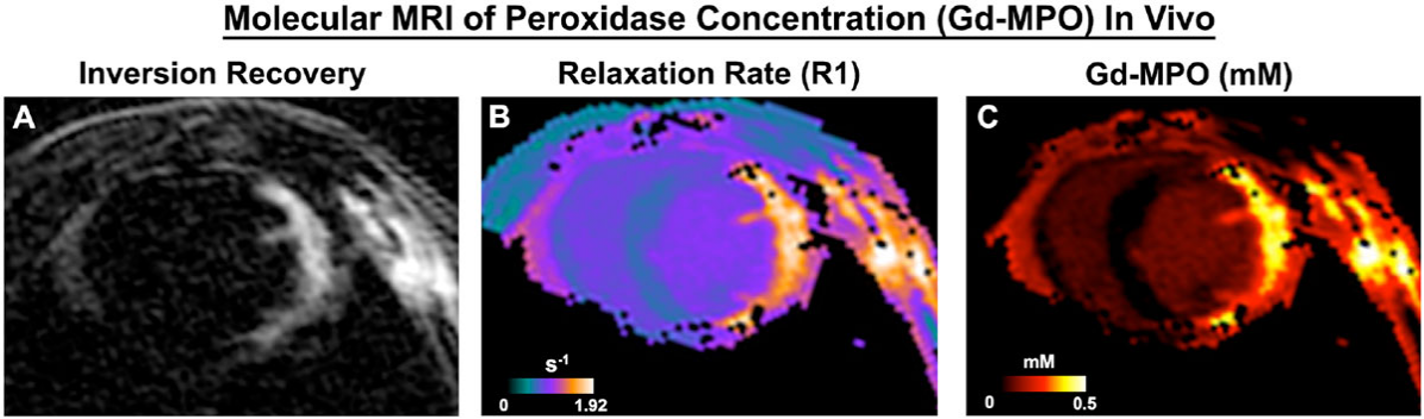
Molecular MRI of Gd-MPO activation in vivo. A. Inversion recovery image 2 hours after probe injection. Unbound Gd has been washed out but activated probe is retained in the ischemic myocardium. B. R1 map of the same slice. C. Quantitative map of Gd-MPO concentration and activity in vivo.
